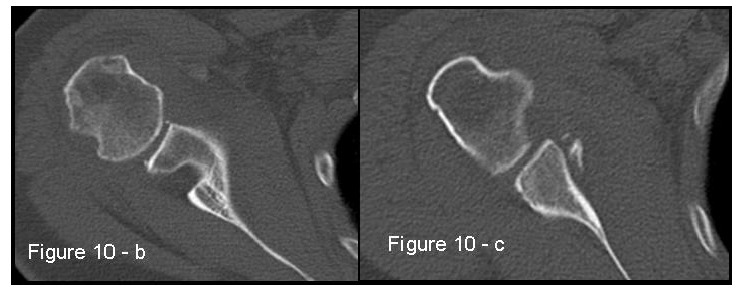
CT is the gold standard for the assessment of bipolar bone defect: b) Hill-Sachs axial view - c) antero-inferior glenoid margin defect axial view. 121x46mm (150 x 150 DPI).
